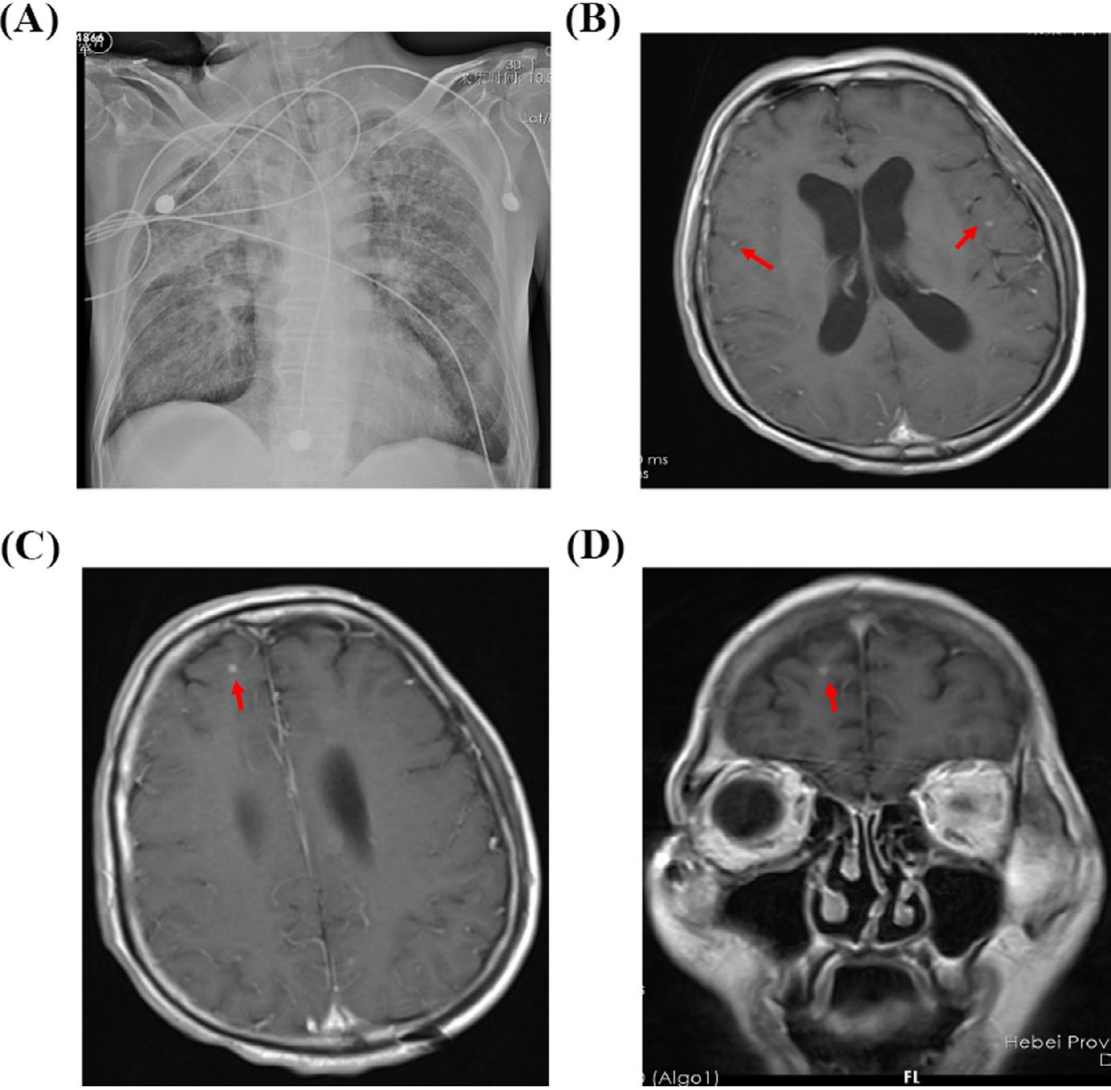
Chest X‐ray and cranial magnetic resonance imaging (MRI) of the patient with tuberculous meningitis. A, Chest X‐ray showed multiple patches and nodules with observable cavity in his lungs. B‐D, T1‐weighted image showed enhanced nodules in gray matter and white matter border at the right frontal region, as well as lateral meningeal enhancement at the left temporal lobe
